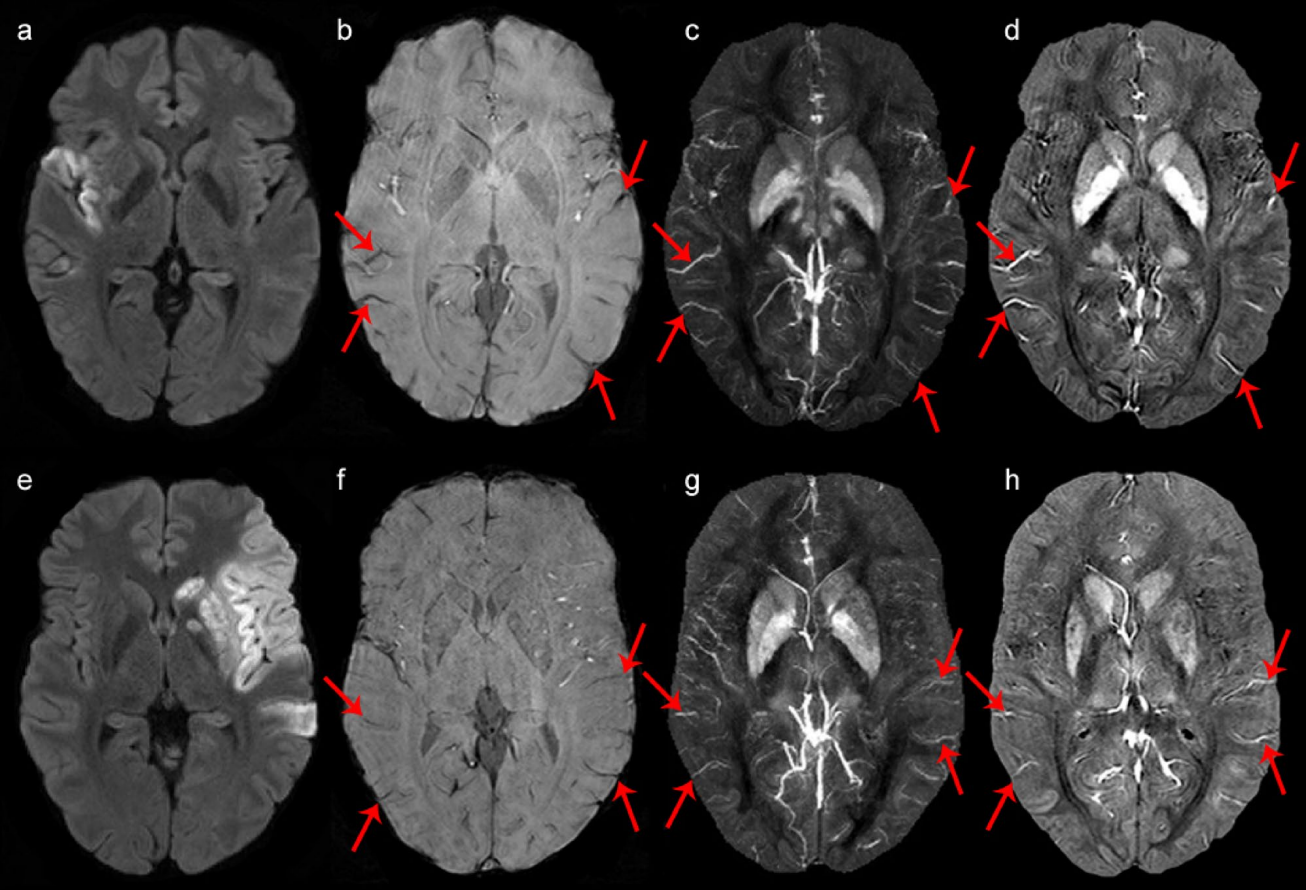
Comparison of DWI, SWI, MIP and QSM in 2 different patients for cortical vein identification. (a–d) represent one patient while (e–h) represent another patient. Image (a) and (e) demonstrate the ischemic core of the ischemic hemisphere, which is hyperintense on DWI. A selection of cortical veins (red arrows) is demonstrated on SWI (b and f), MIP (c and g) and QSM (d and h) in control and ischemic hemisphere. Note that the visibility of cortical veins within the infarction core is reduced. Cortical veins within the ipsilateral non-ischemic MCA territory and contralateral MCA territory seem to have comparable intensity.
